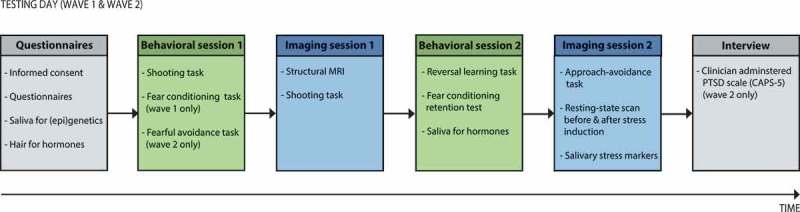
Overview of the testing days. The wave 1 and wave 2 assessments consist of similar testing procedures, except for two minor differences in Behavioural session 1 and the Interview (see figure). MRI = magnetic resonance imaging.
